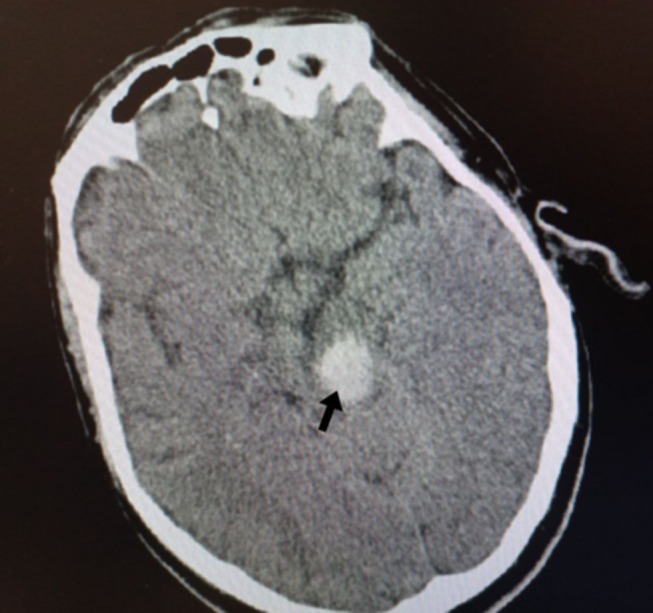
Axial computed tomography scan of the brain shows relatively hyperdensity in unilateral mesencephalic in favor of hemorrhage (arrow).
